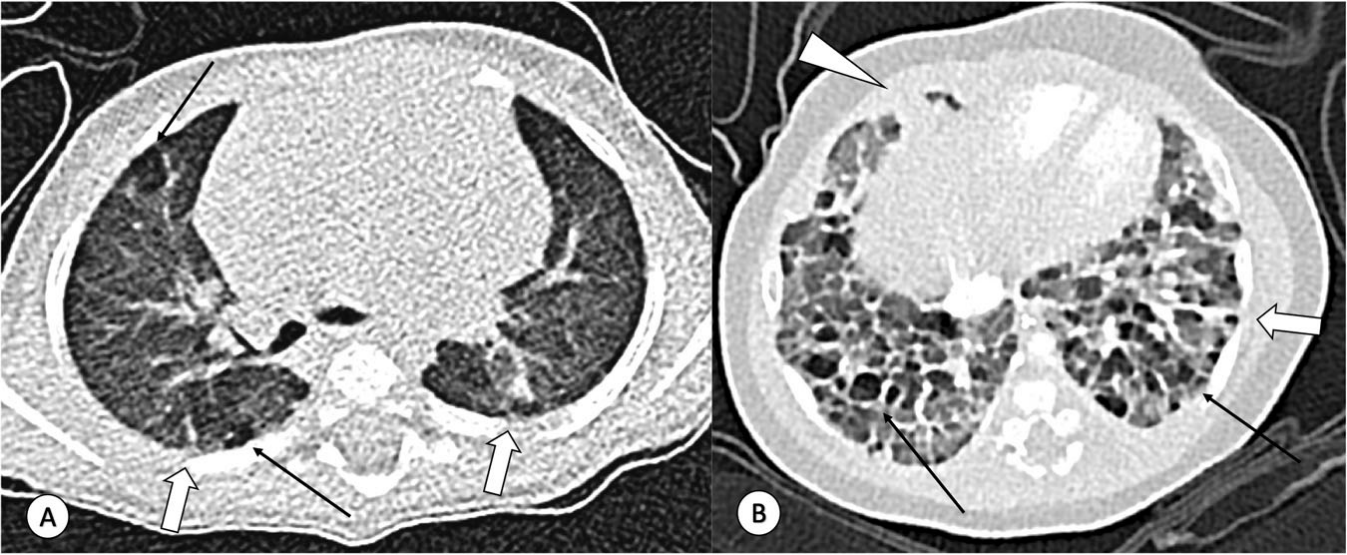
Bronchopulmonary dysplasia (BPD): A Free-breathing CT image of a mild BPD patient and (B) Photon Counting CT image of a severe BPD patient. Typical features of BPD include areas of low attenuation (arrows) observed in both mild and severe BPD cases, linear opacities (thick arrow), and consolidation (arrowhead) in severe BPD patients
